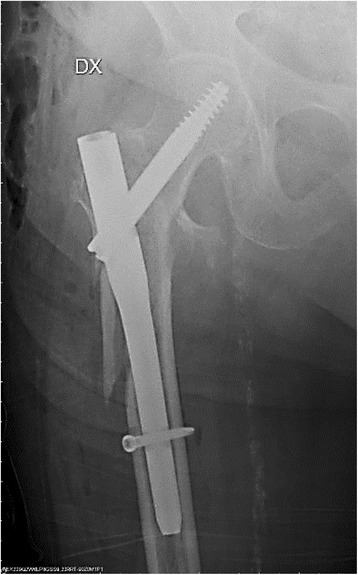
Radiograph of Zimmer nail with cephalic screw with TAD lower than 25 mm for 31-A3
